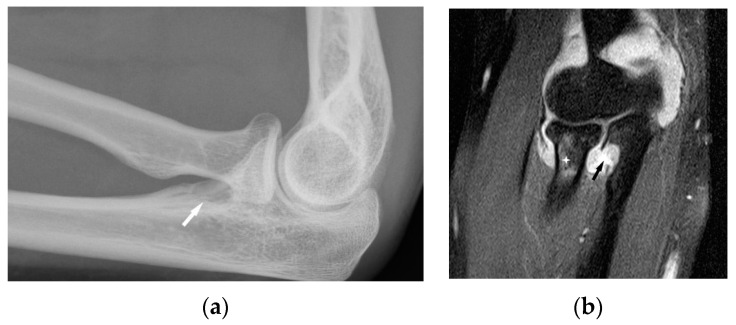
Radiograph (a) and MRI of the elbow (b) performed in a 45-year-old woman with sarcoidosis. Osteolytic lesion in the proximal part of the elbow bone ((a), white arrow). T2-weighted image with fat saturation revealed a synovitis, erosion in the proximal part of the elbow bone corresponding to this bone lesion (black arrow) and slight bone marrow edema in the head and neck of the radius (asterisk).
